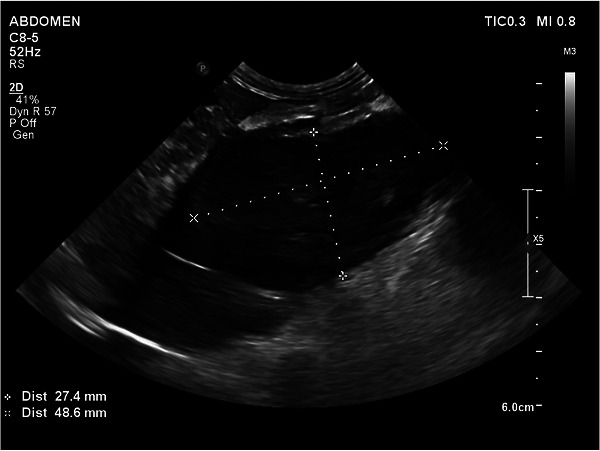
Abdominal ultrasonography after 2 months of therapy. The cranial abdominal mass measured approximately 4.9 × 2.7 cm, markedly reduced compared with the initial study, indicating partial therapeutic response.
